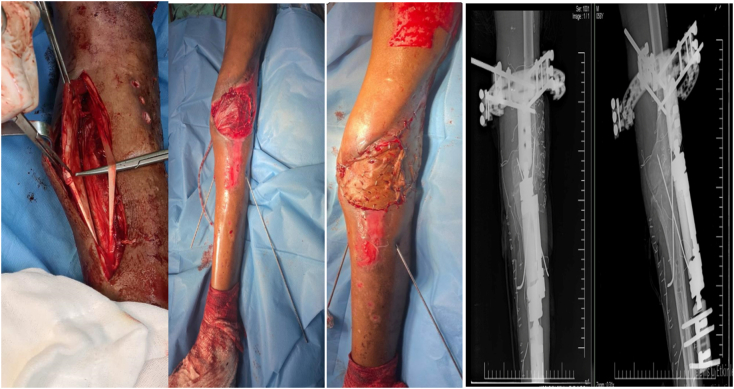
Intraoperative medial gastrocnemius flap elevated and transposed to reconstruct the defect. Full thickness skin graft used to cover muscular belly. Anteroposterior and lateral radiographs showing reduction of the joint and fixation with hybrid external fixator augmented by two crossed Steinmann pins.
